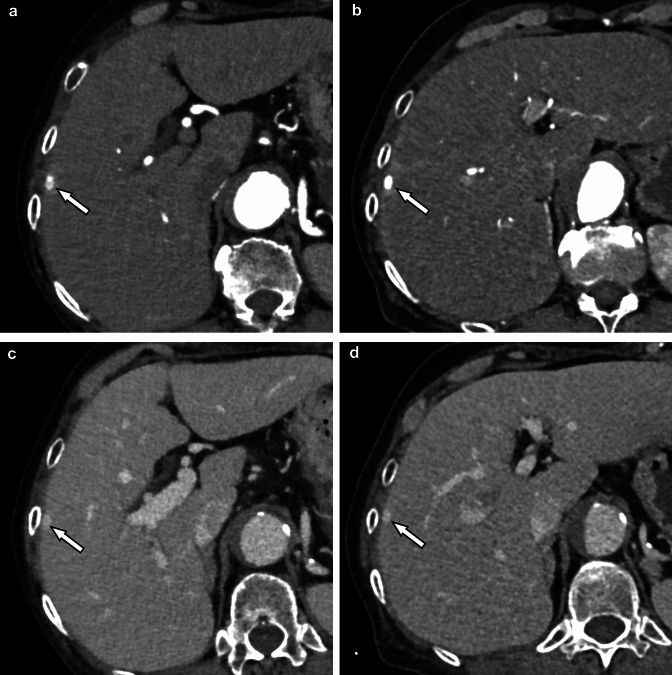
Contrast-enhanced dynamic CT images during the arterial dominant phase (A, B) and the equilibrium phase (C, D) of the liver scanned with photon-counting CT (A, C) and conventional dual-source CT (single-energy mode) (B, D) on different days. A small hemangioma is seen under the capsule of the right lobe of the liver (arrows). Although the radiation dose of the photon-counting CT (CTDI_vol_: 4.6 mGy) was coincidently half of that of the conventional CT (9.2 mGy), the image quality remained comparable
